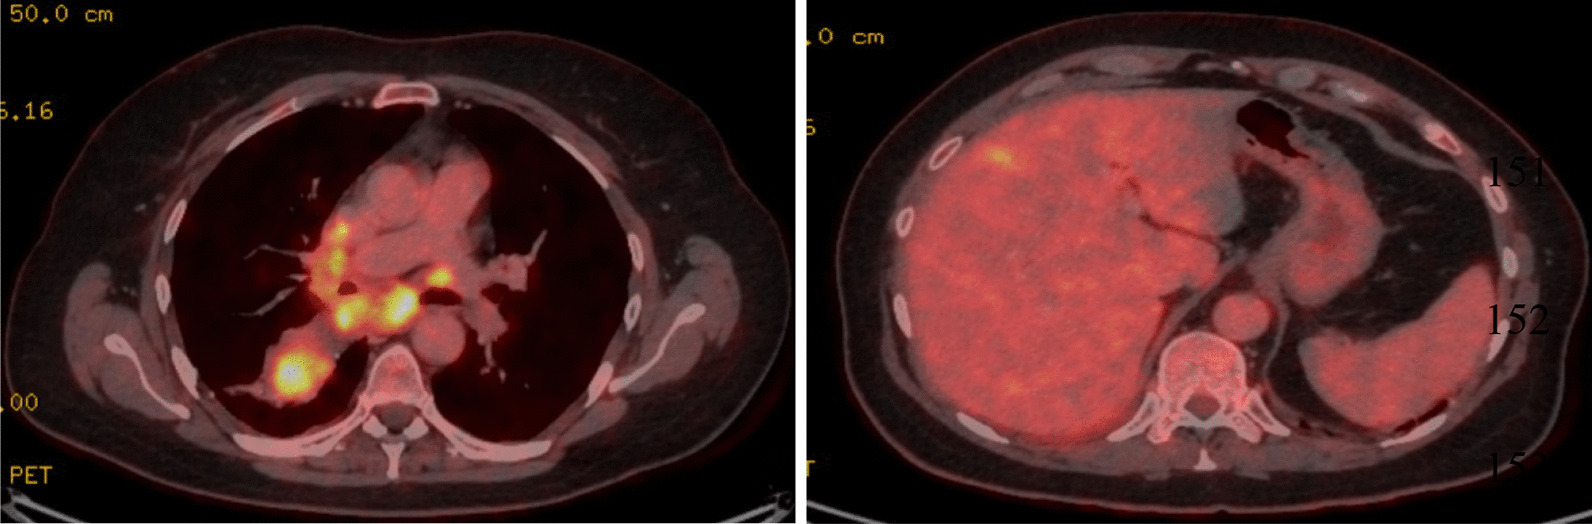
Positron Emission Tomography-Computed Tomography (PET-CT) at presentation
